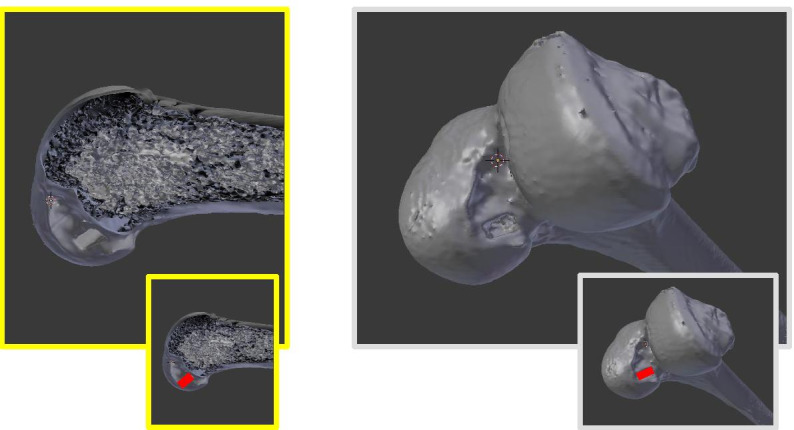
CT scans with three-dimensional image reconstructions of the femoral tunnel performed with a specific guide
